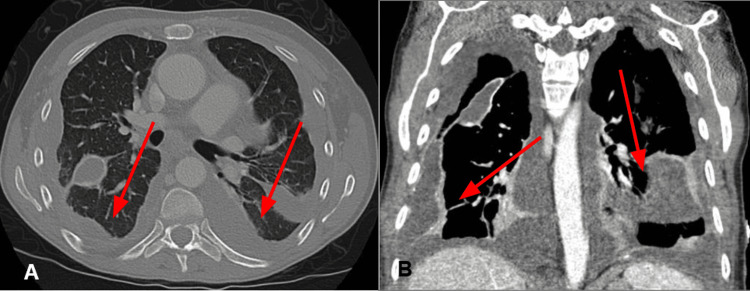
Post VATS CT scan Transverse (A) and coronal (B) view revealing improved and redistributed bilateral pleural effusions (red arrows). VATS: Video-assisted thoracic surgery.
